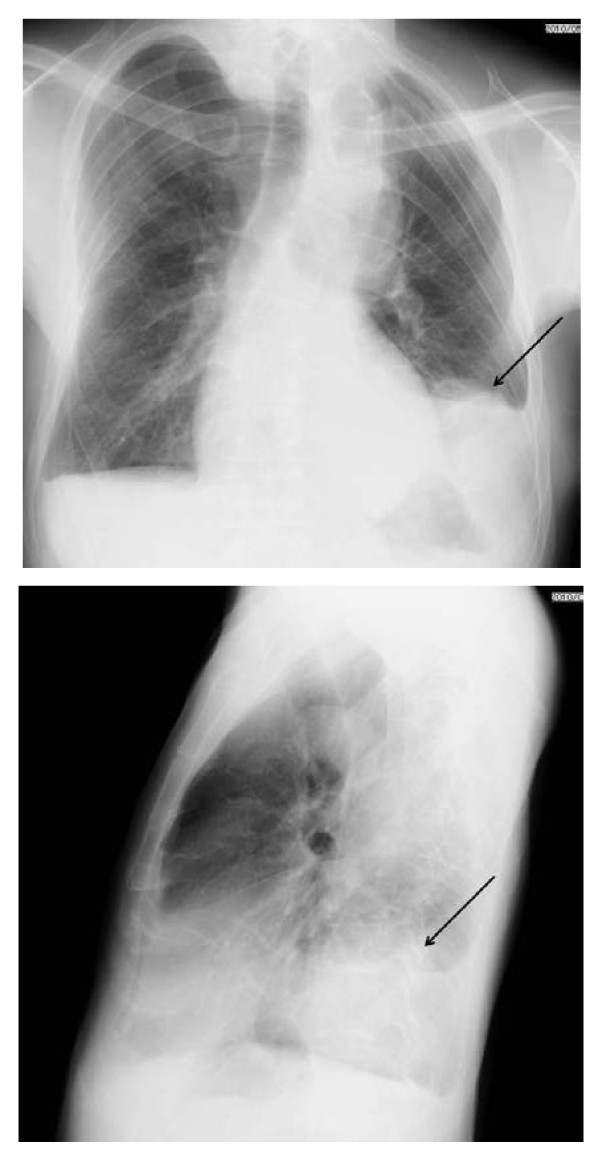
Chest Xray. Chest Xray shows severe scoliosis and large abnormal shadow (arrows) in left infero-posterior chest.
